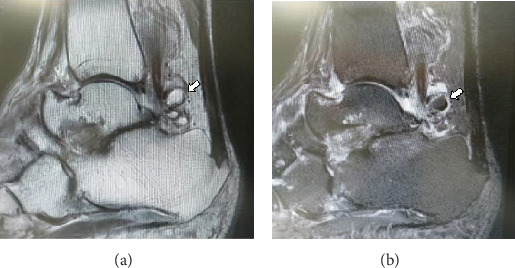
Magnetic resonance imaging shows several small fusiform masses (arrow) surrounded by a fluid signal on T2-weighted (a) and short T1 inversion recovery (b) scans in the sagittal plane.
